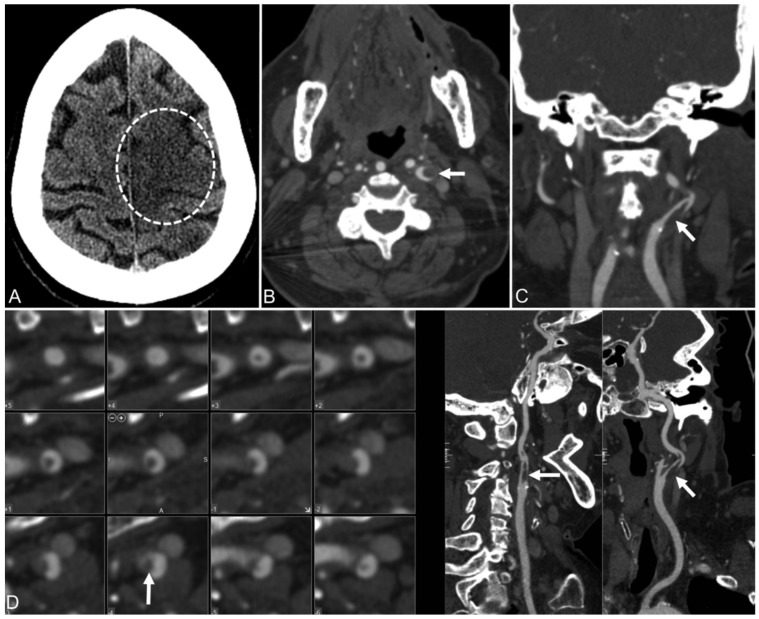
Patient 2. (A) 78-year-old woman with COVID-19 and an NIHSS score of 25.The CT of the head without comparison shows an evolving ischemic infarct in the left frontal brain paracentral cortex (dotted circle) and a smaller infarct in the left parietal cortex. (B–D), Axial, coronal, and curved reimaged images from CT angiography of the head and neck show an irregular plaque at the left internal carotid artery bifurcation and a capillary filling defect (arrow) extending superiorly in the left internal carotid artery, which matches the ruptured plaque with clot formation [144].
